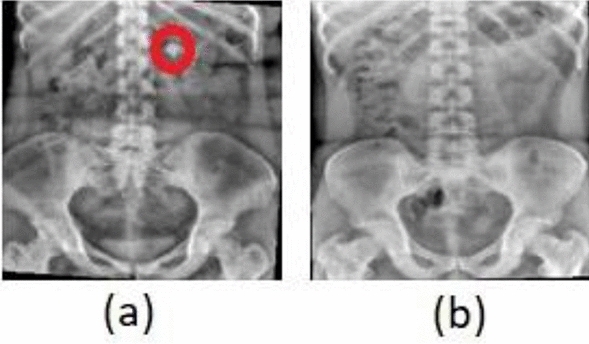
KUB x-ray sample images: (a) Kidney Stone; (b) Normal.
